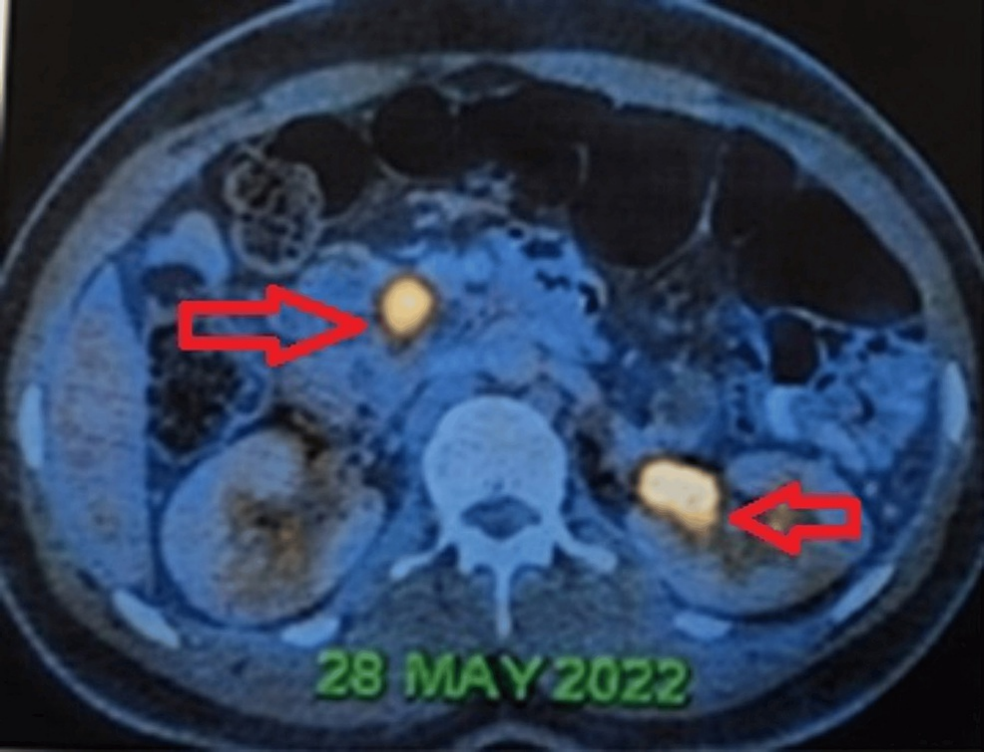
Positron emission tomography-computed tomography image showing cystic lesions in the pancreas, with lesions having increased metabolic activity in the head/uncinate process of the pancreas and interpolar region of the left kidney.
